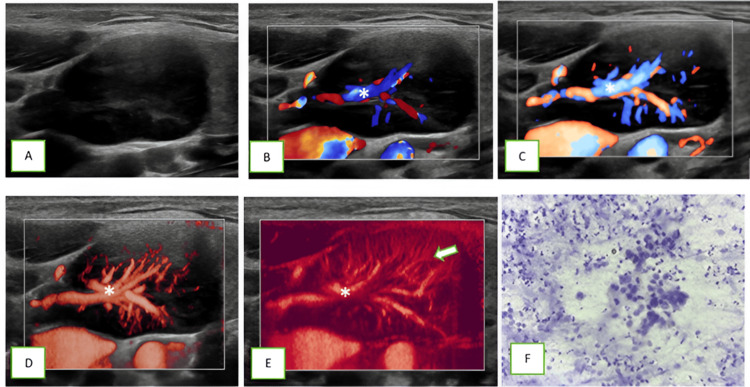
Reactive lymphadenitis. A 35-year-old woman presented with fever, mild throat pain, and bilateral palpable cervical lymph nodes. (A) Gray-scale ultrasound showed a poorly visualized hilum. (B-E) Color, power Doppler, MVI (color), MVI (power), respectively, demonstrated prominent hilar vessels (*) with enhanced detection of small vessels by MVI, highest by MVI (power mode) (white arrow)-suggesting an inflammatory etiology. (F) Corresponding cytology showed reactive lymphoid cells consistent with reactive lymphadenitis. MVI: microvascular imaging
